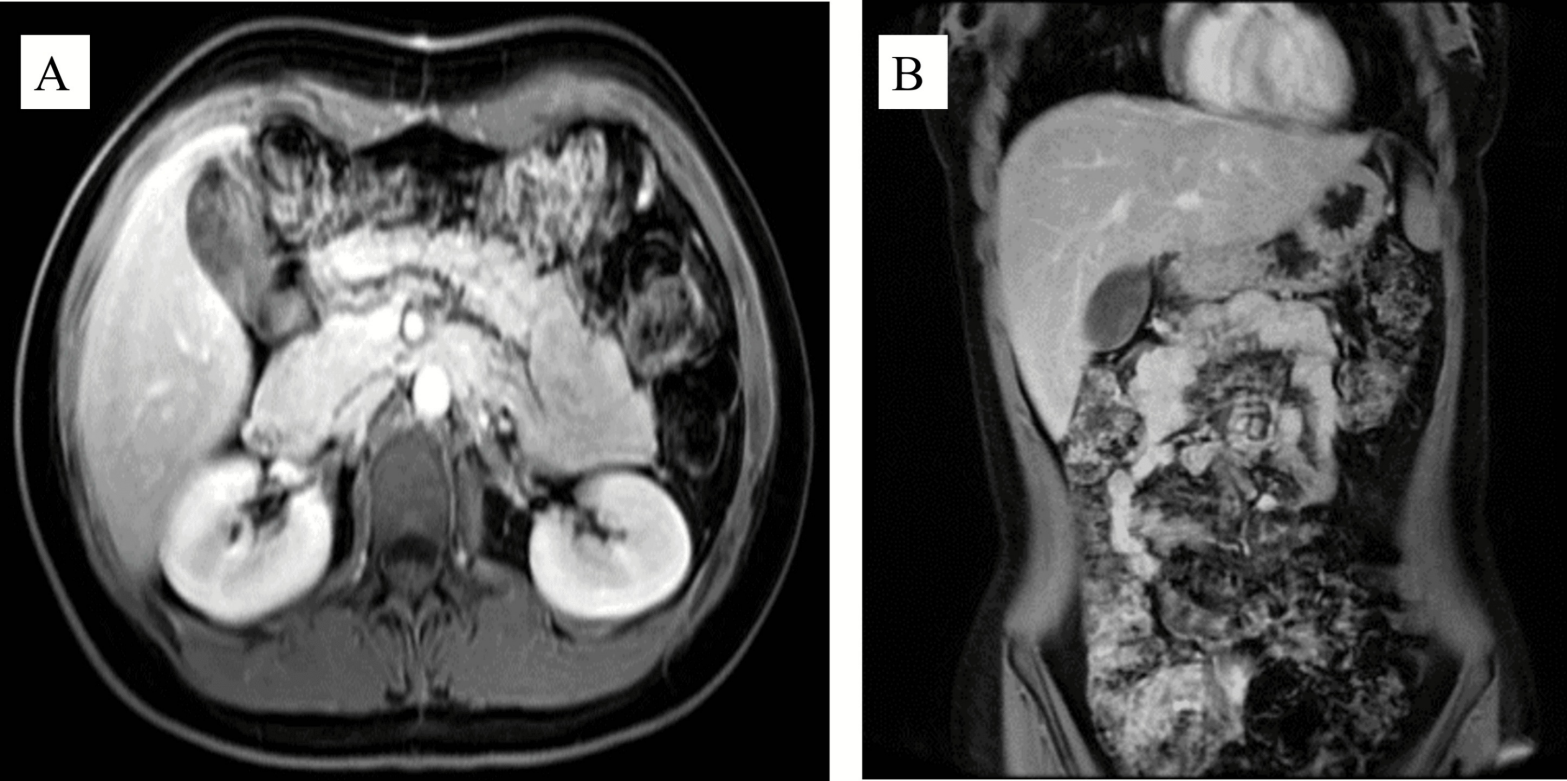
MRI of the abdomen after surgery. A: Axial T1-weighted post-contrast MRI image of the abdomen. Follow-up post-surgical excision of solid pseudopapillary neoplasm of the pancreas showing postsurgical changes in the pancreatic bed with no evidence of residual or recurrent mass. Surrounding abdominal structures appear unremarkable in this slice. B: Coronal T2-weighted MRI image of the abdomen: Follow-up post-surgical excision of solid pseudopapillary neoplasm of the pancreas, demonstrating postsurgical changes in the pancreatic bed with no evidence of residual or recurrent mass within the surgical site.
